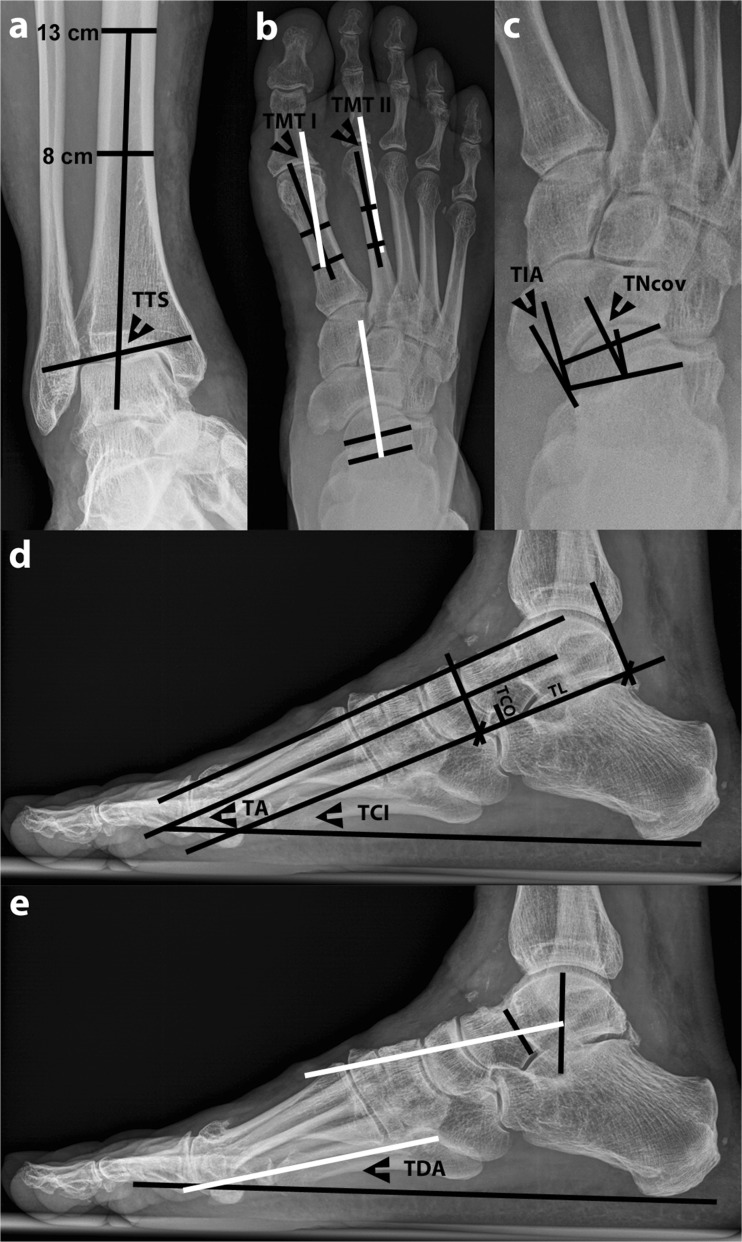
Weight-bearing radiography of a patient with varus ankle osteoarthritis showing the radiographic measurements to determine the three-dimensional position of the talus. The following measurements were performed: a Frontal tibiotalar surface angle (TTS): the mid-longitudinal tibial axis was formed by a line bisecting the tibia at 8 and 13 cm above the tibial plafond. b Horizontal talometatarsal I and II angles (TMT I and II): all* white lines* run parallel and represent the talar axis. c Talonavicular coverage angle (TNcov) and talonavicular incongruency angle (TIA). d Talar angle (TA), talocalcaneal inclination angle (TCI), talocalcaneal overlap (TCO), and talar length (TL). e Talar declination angle (TDA): both* white lines* run parallel and represent the talar axis. The tarsal index in d was determined by using TCI, TCO, and TL
